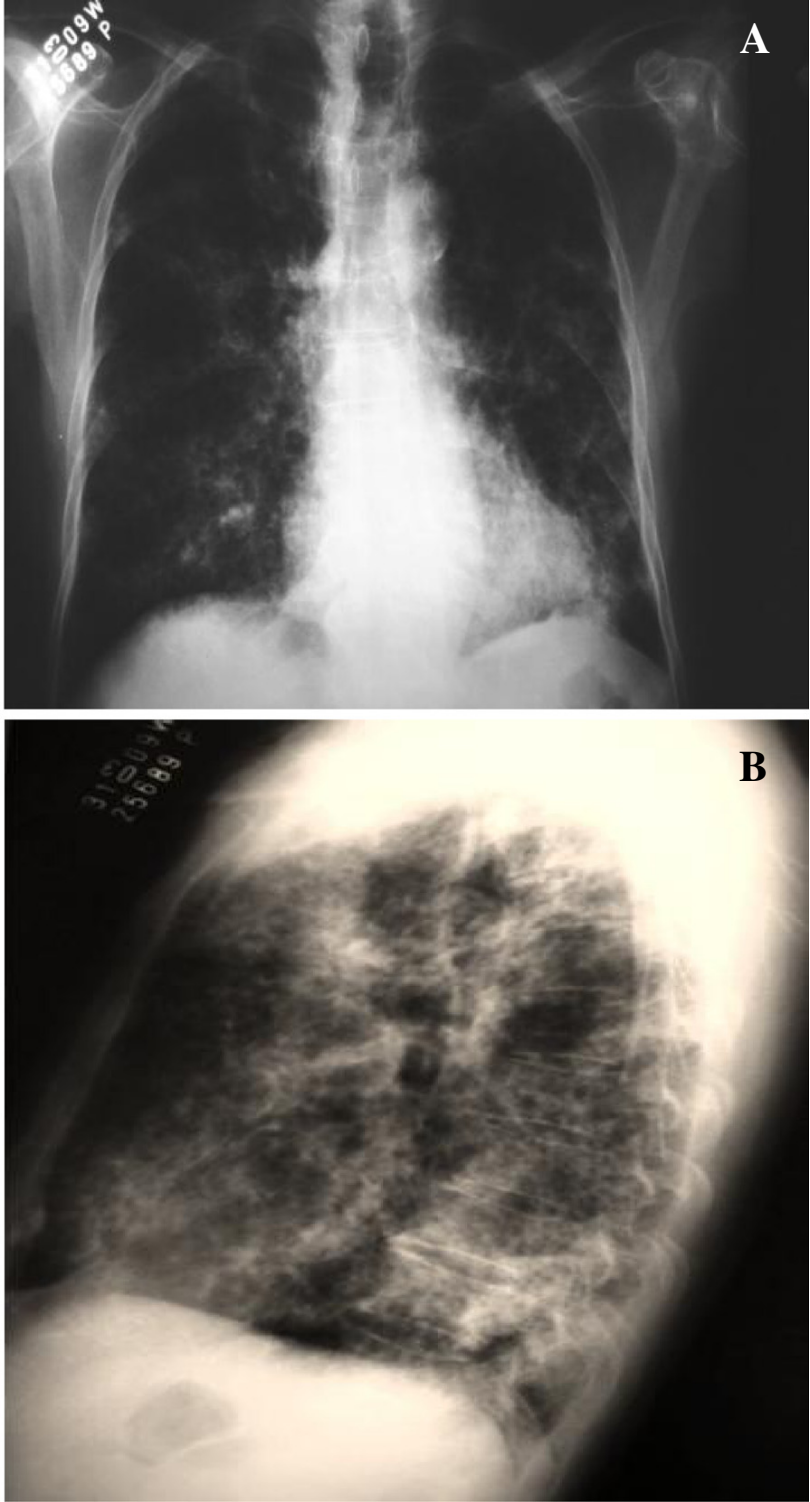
Radiography of the thorax. Anteroposterior view (A) and lateral view (B) showing bilaterally diffuse nodular reticular interstitial lesions.
